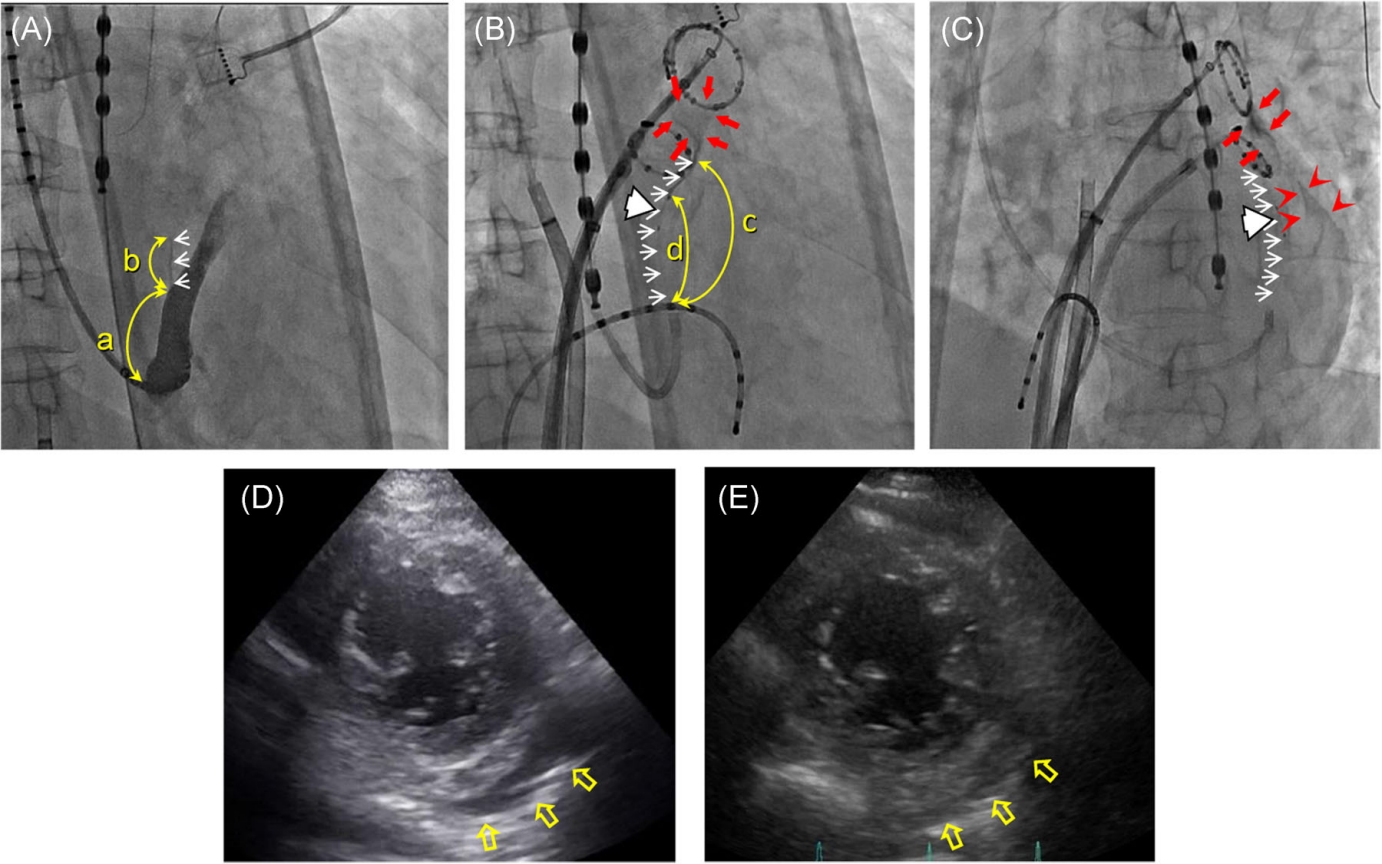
RAO (A,B) and left anterior oblique (LAO) (C) views of the procedural series and echocardiography (D,E) of a representative case with documented apparent leakage of contrast medium into the pericardial space during the initial injection, but the pericardial effusion had almost completely resolved by the next day (63‐year‐old female, 155.0 cm, 52.5 kg, with no structural heart disease). A, CS venography without an occlusion balloon. B,C, The initial selective VOM‐graphy via the balloon lumen in the RAO (B) and LAO (C) views. D,E, Short axis views of the echocardiography examined soon after the session (D) and on the next day (E). Note that fan‐shaped leakage of the contrast medium (red arrows) (B,C) and contrast retention along the cardiac silhouette (red arrowhead) (C) are observed. The pericardial effusion is observed outside of the relatively thick adipose layer (yellow empty arrows) soon after the session despite no deterioration of her hemodynamic condition (D), which becomes mostly resolved by the next day (E). The values of the parameters measured regarding the VOM features were as follows: a = 25.9 mm, b = 14.51 mm, c = 41.74 mm, and d = 30.49 mm. Abbreviations, (a) through (d), the white arrows, and white arrowheads indicate the same as in Figure 2
